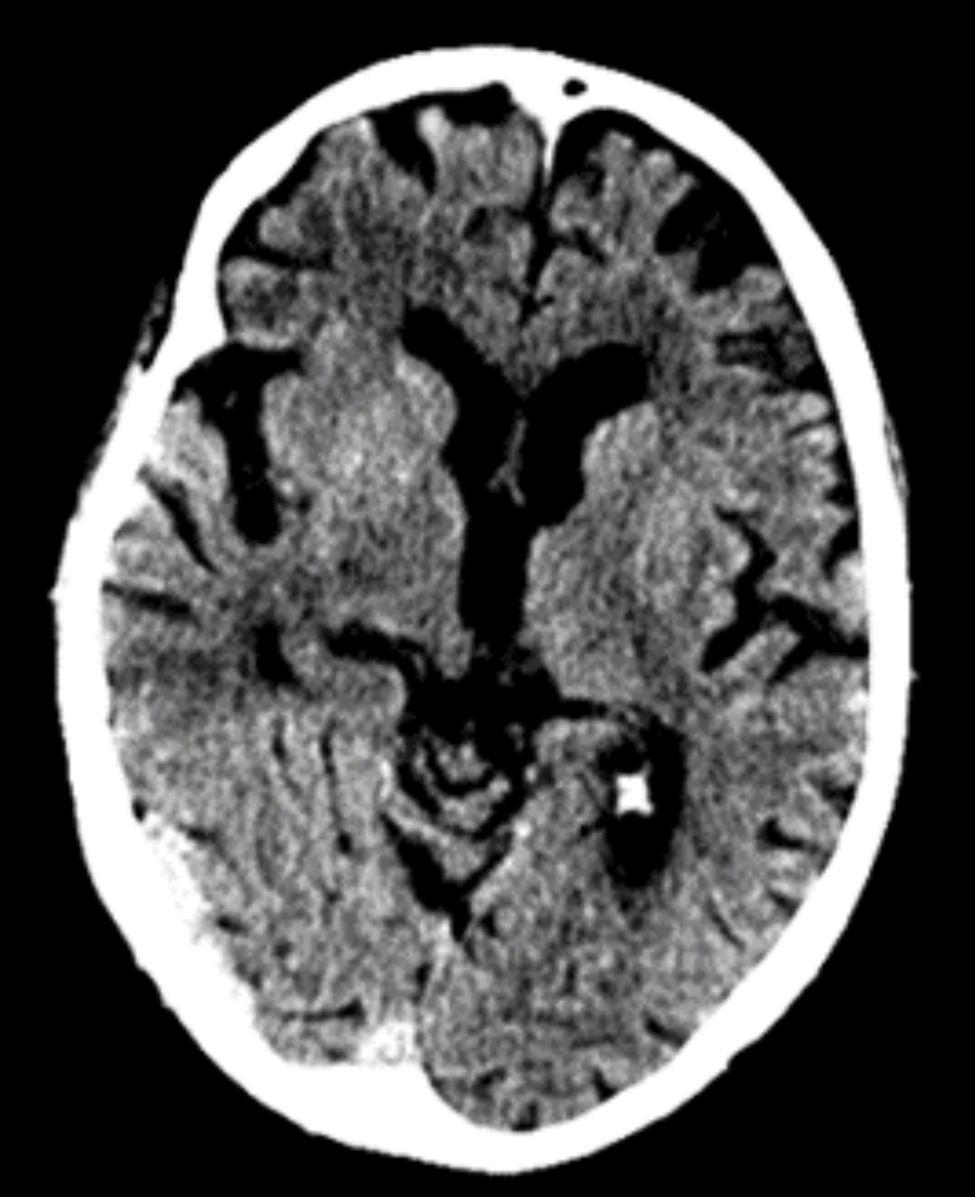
Axial CT brain scan showing an ASPECTS score of 10 with no evidence of acute ischemic or hemorrhagic vascular lesions CT: computed tomography, ASPECTS: Alberta Stroke Programme Early CT Score
